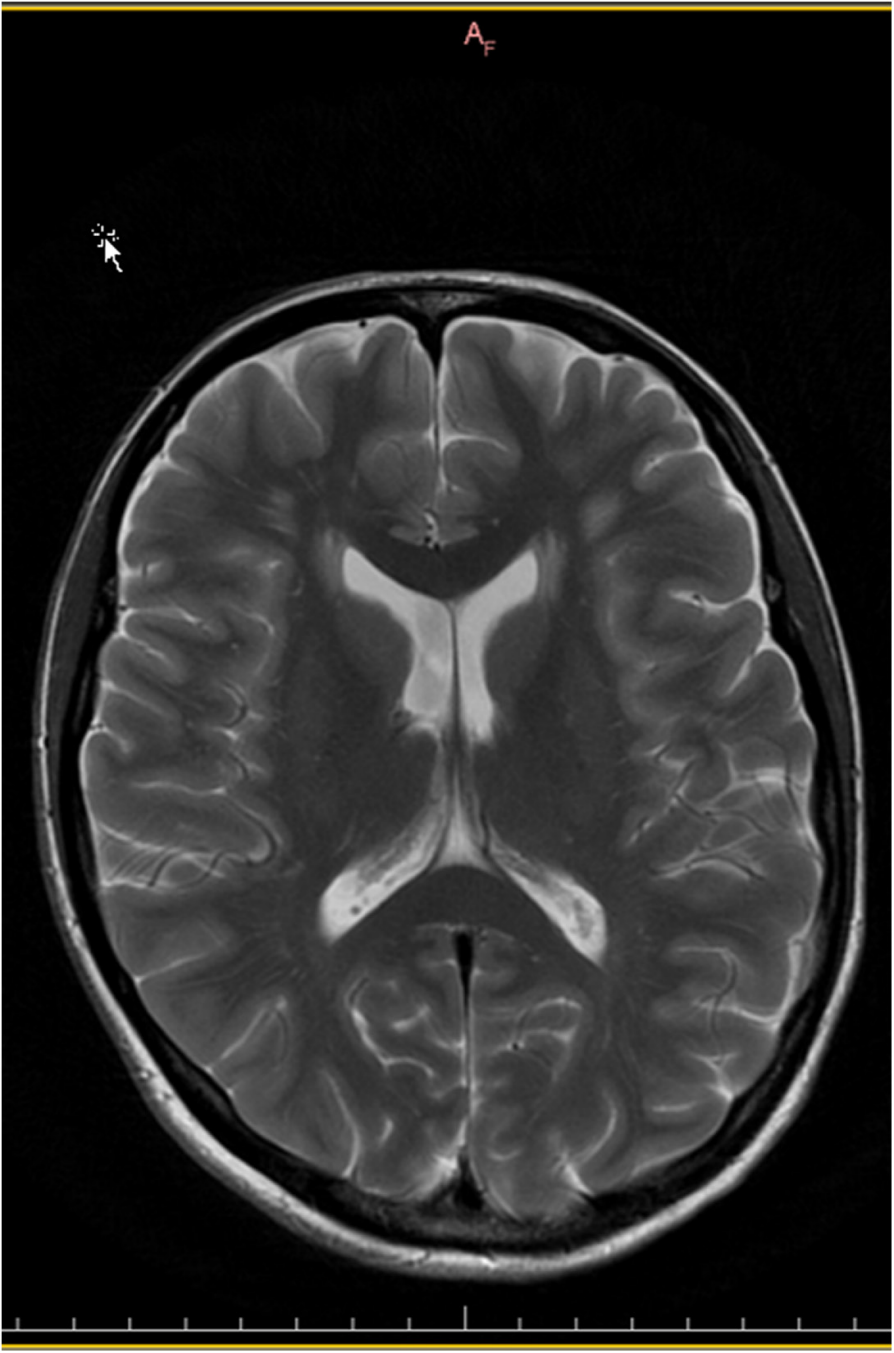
Cranial MR imaging shows symmetric periventricular white matter hyperintensities on T2‐weighted studies and slightly hypointensive signal relative to adjacent brain on T1‐weighted studies. No ventricular enlargement or mass effect was present
